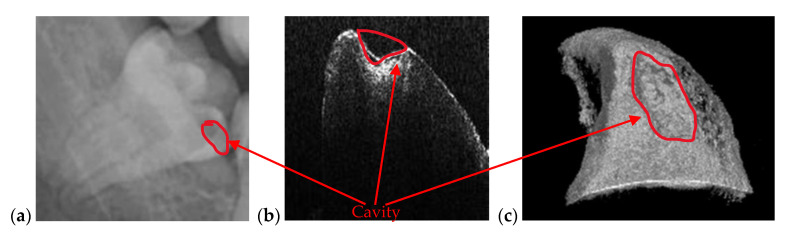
(a) Cavity of a third molar from the fourth quadrant assessed on a section cropped from a panoramic radiography. OCT investigation on the tooth extracted for medical purposes after performing the radiography: (b) B-scan and (c) 3D reconstruction. Patient E.M., male, age 23 years, diagnosed with a cavity on the smooth surface (lateral side) of the third molar, with the following remarks on the clinic condition: the cavity appeared because the third molar is not in a correct position and in that area, between the second and the third molar, the patient cannot perform a full cleaning of the tooth.
